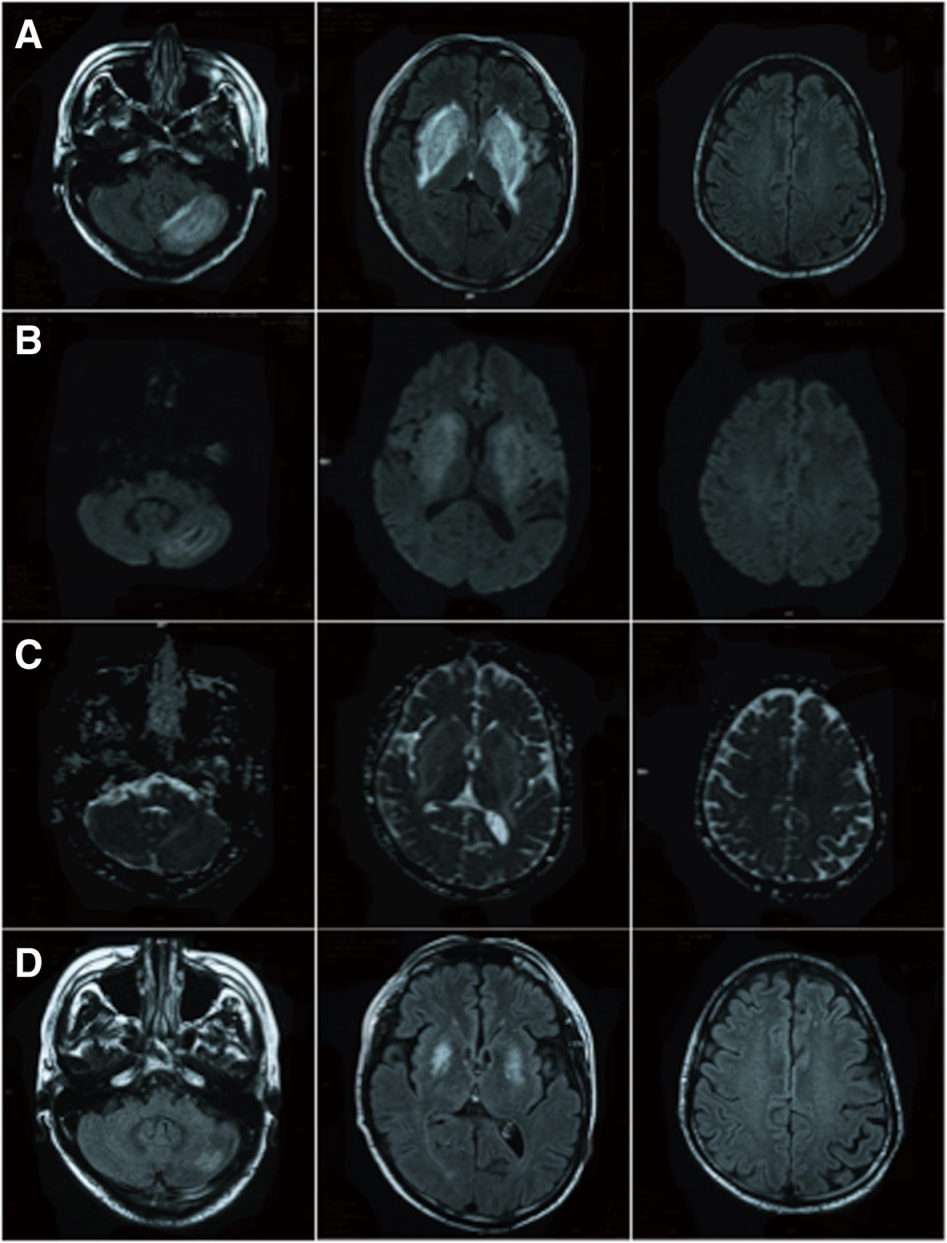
MR images at 1 and 4 weeks after admission. Representative FLAIR images (A), DWI (B), and ADC map (C) at 1 week after admission, and FLAIR images (D) at 4 weeks after admission.
